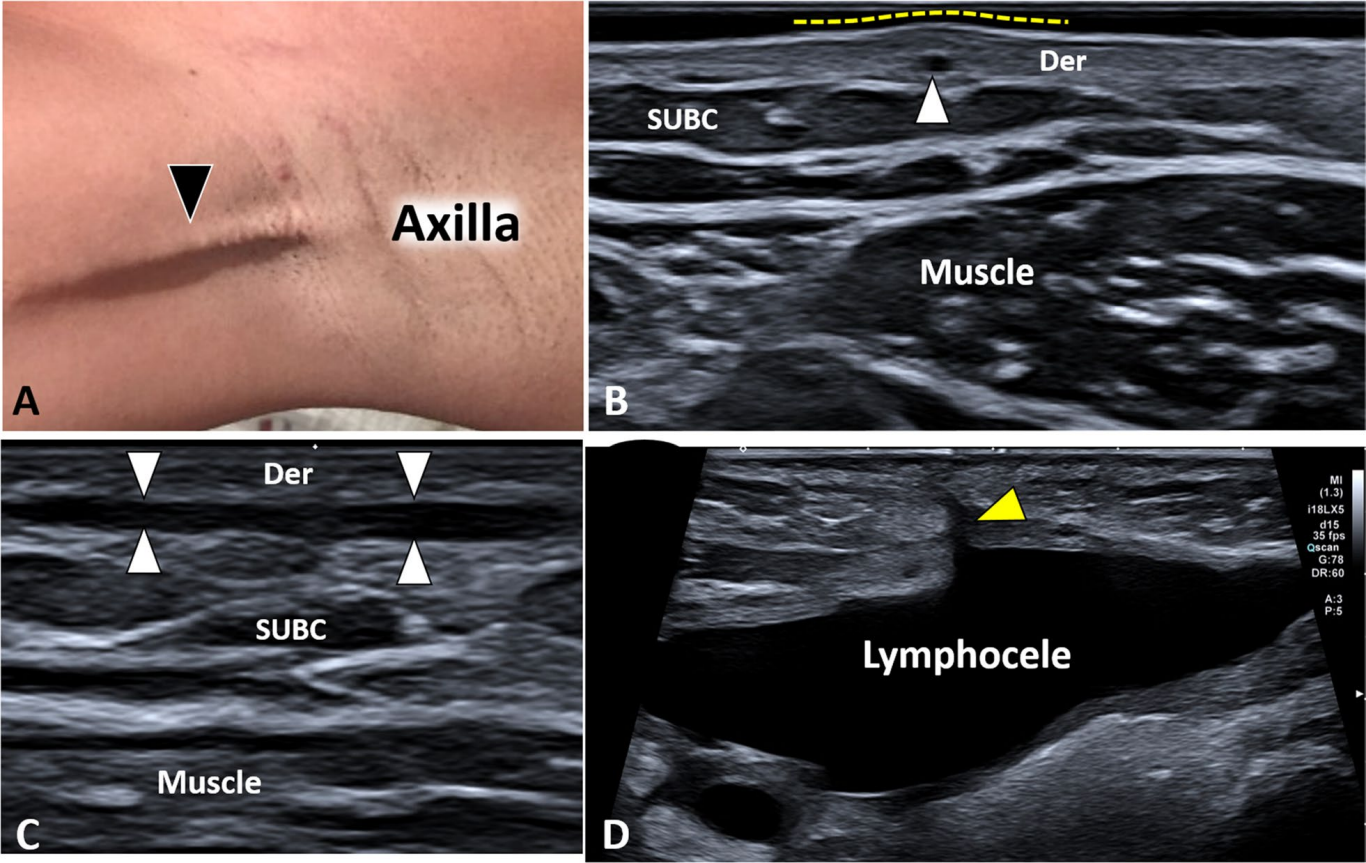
Axillary web syndrome and lymphocele. Axillary web syndrome (A) is clinically characterized by very superficial, painful and retractile cords *(black arrowhead)*. Transverse (B) and longitudinal (C) sonogram—using a high-frequency probe—shows the hypoechoic band *(white arrowheads)* coursing within the dermal layer *(Der)* of the axillary region. Of note, a large amount of gel allows a prompt identification of the skin bulging *(yellow dotted line)* where the lymphatic cord *(white arrowhead)* should be sonographically assessed (B). A large lymphocele (D) presents as anechoic irregular-in-shape fluid collection and its peduncular connection *(yellow arrowhead)* with the overlying surgical incision is clearly visible as well. SUBC: subcutaneous tissue
